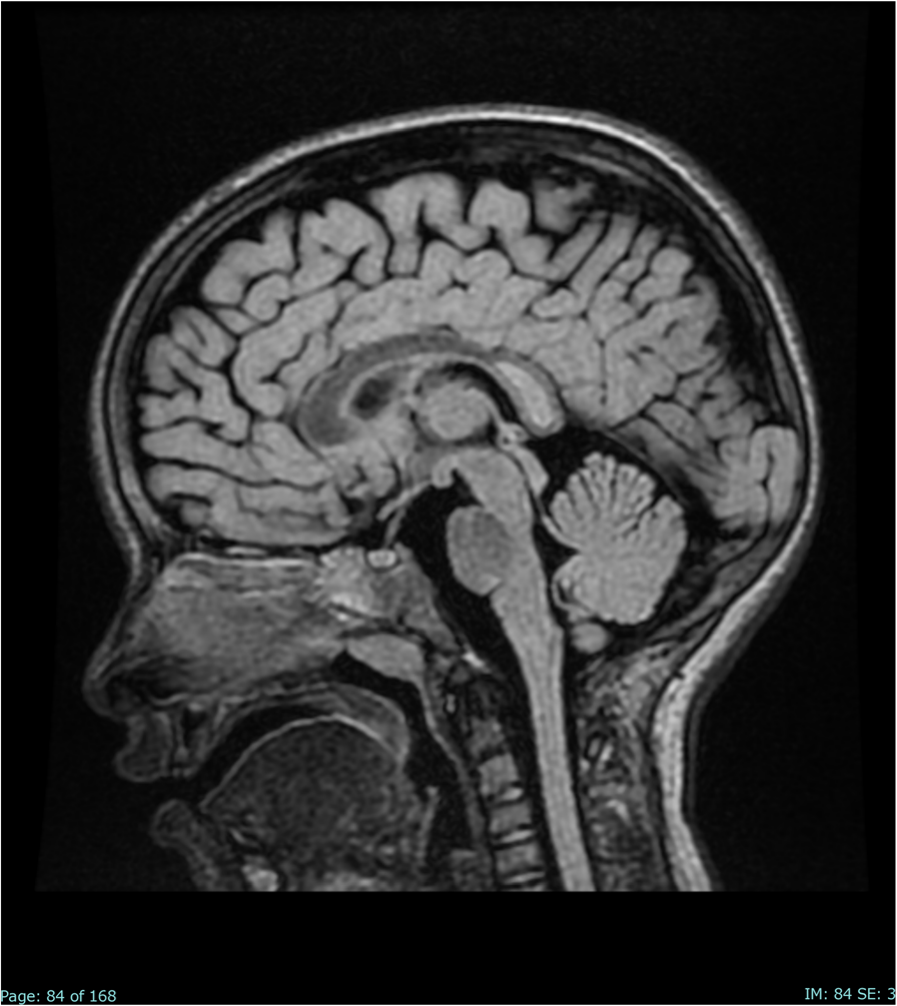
Sagittal FLAIR image on the day of admission shows a focal high-signal lesion in the splenium of the corpus callosum
